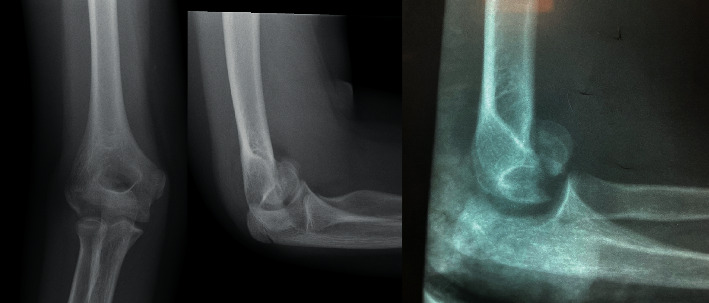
Patient number 1, posttraumatic X-rays, and control lateral view after 3 weeks from injury.
